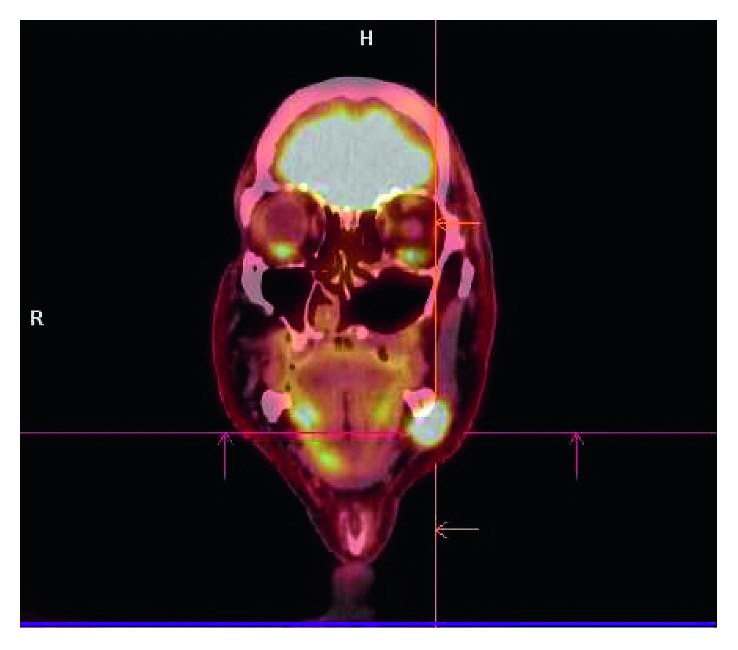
PET-CT shows hypercaptation area of 1.9 cm in the left cervical level I, compatible with metastasis.
